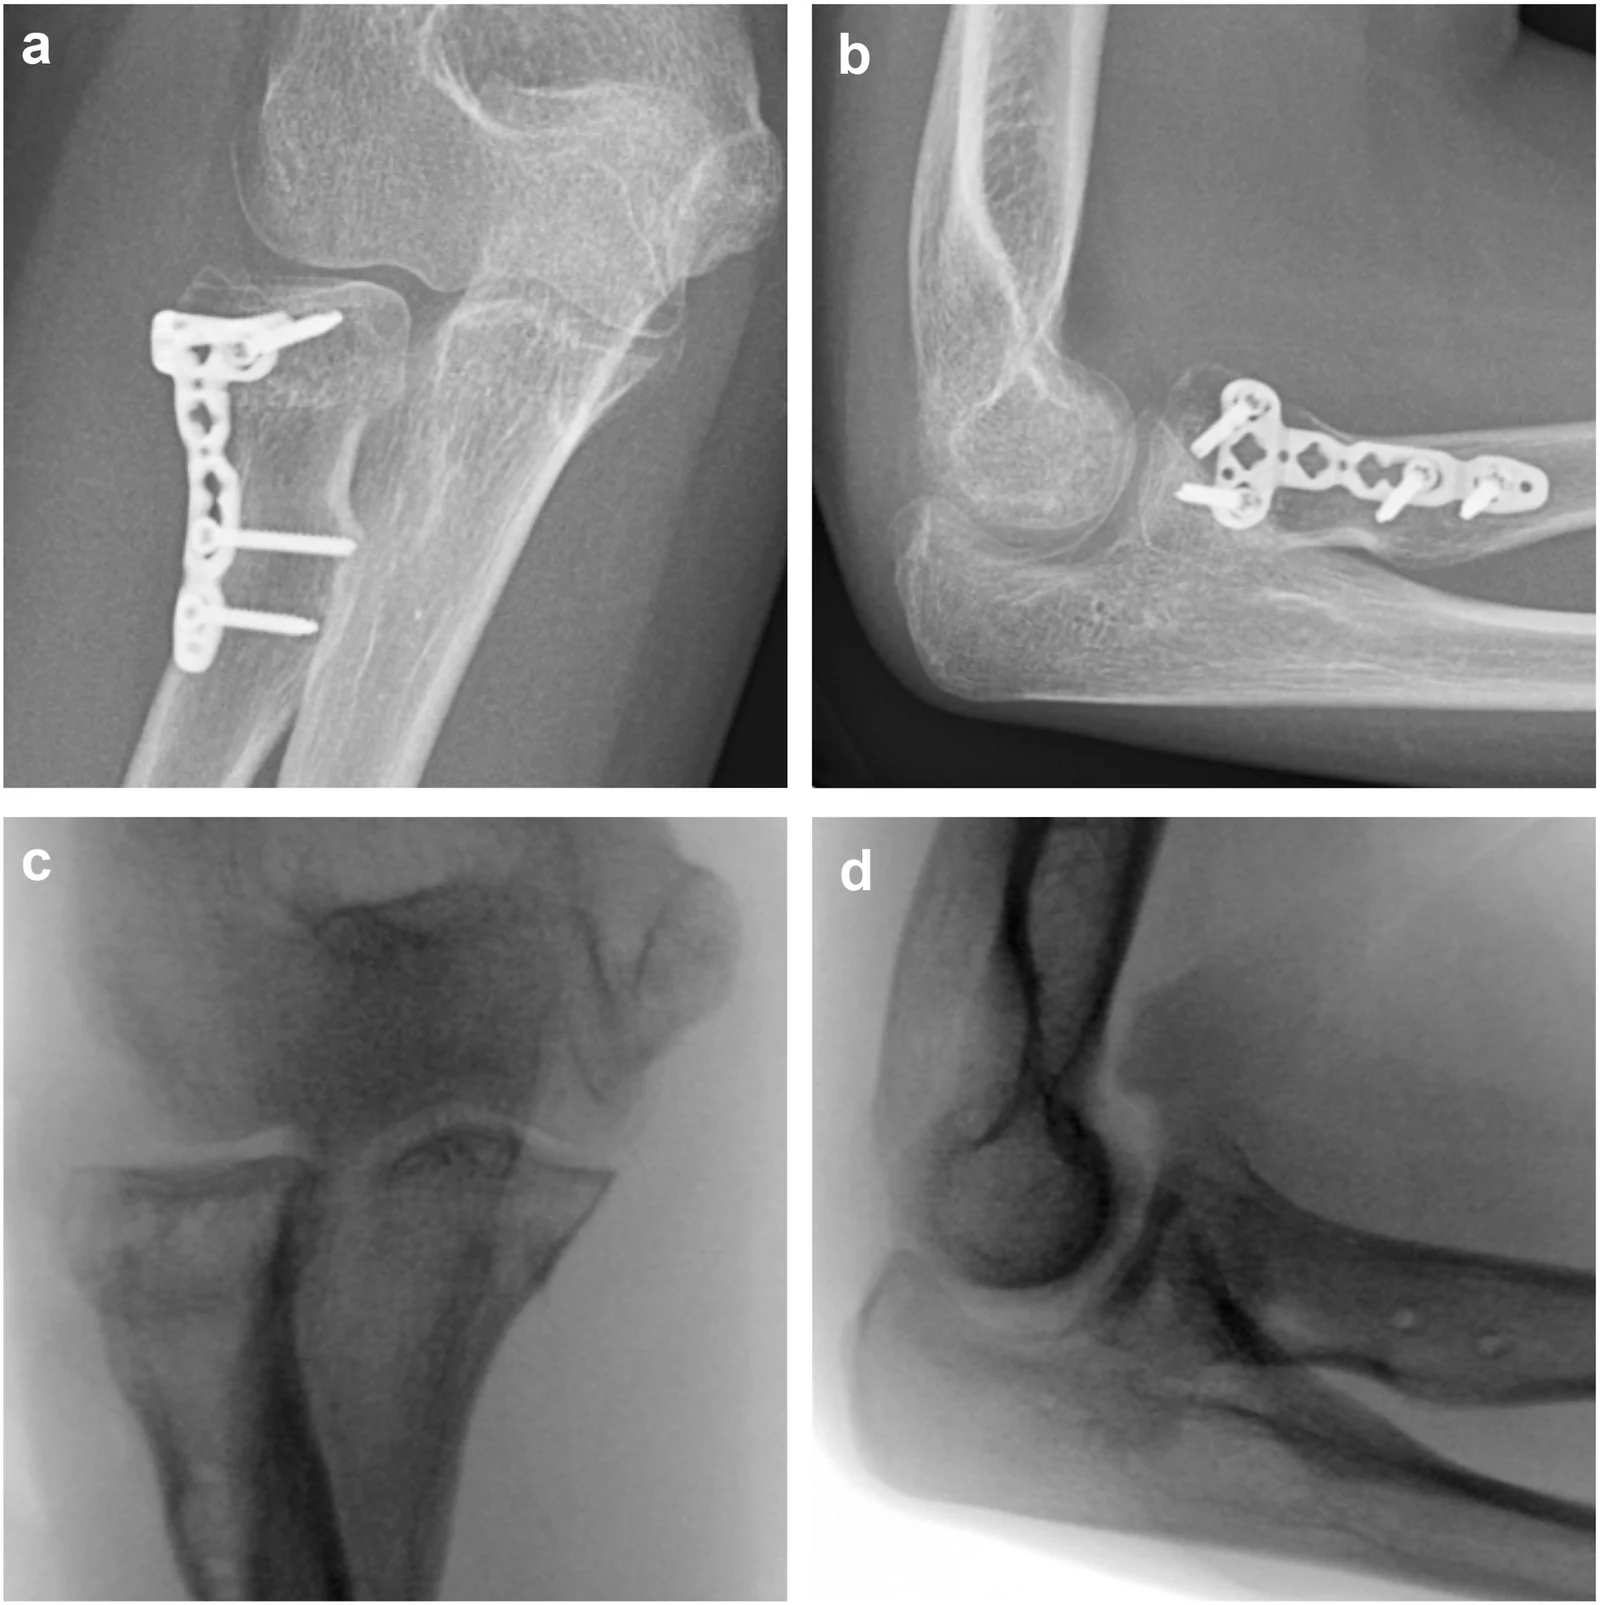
Follow-up radiographs: radiographs 3 months post-operatively (a, b) and radiographs after plate removal (c, d).
